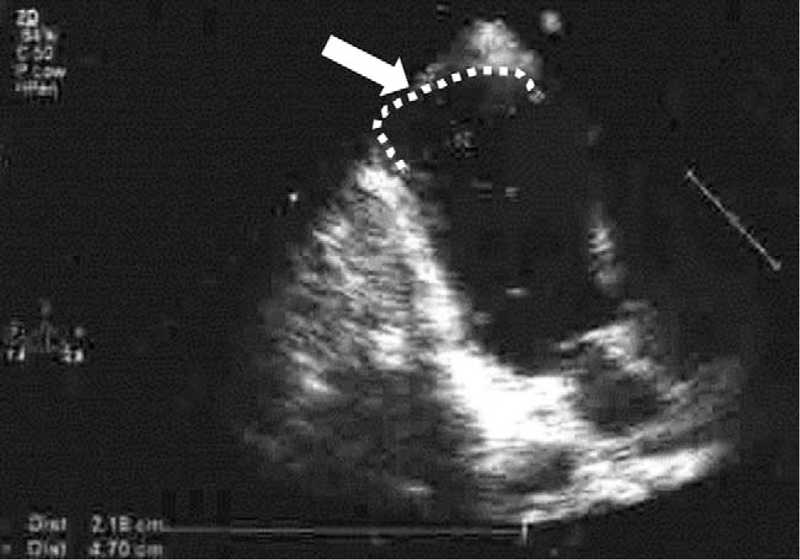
Two-dimensional echocardiographic image in apical in patient #5, 2-chamber view showing a sizable apical aneurysm (arrow).
